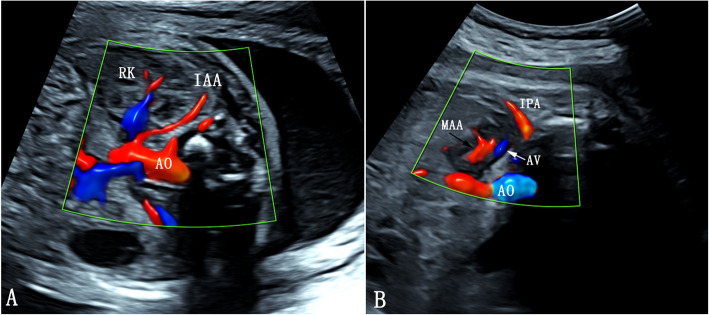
The color Doppler of A) the inferior adrenal artery and B) the middle adrenal artery. a IAA mainly branches obliquely from the proximal segment of the renal artery and then travels along the upper pole of the kidney with a curved course, supplying the posterior and inferolateral outer zone of the gland. b MAA travels along the single central adrenal vein in the medial part of the gland. IAA, inferior adrenal artery; RK, right kidney; AO, aorta; MAA, middle adrenal artery; AV, adrenal vein; IPA, inferior phrenic artery
